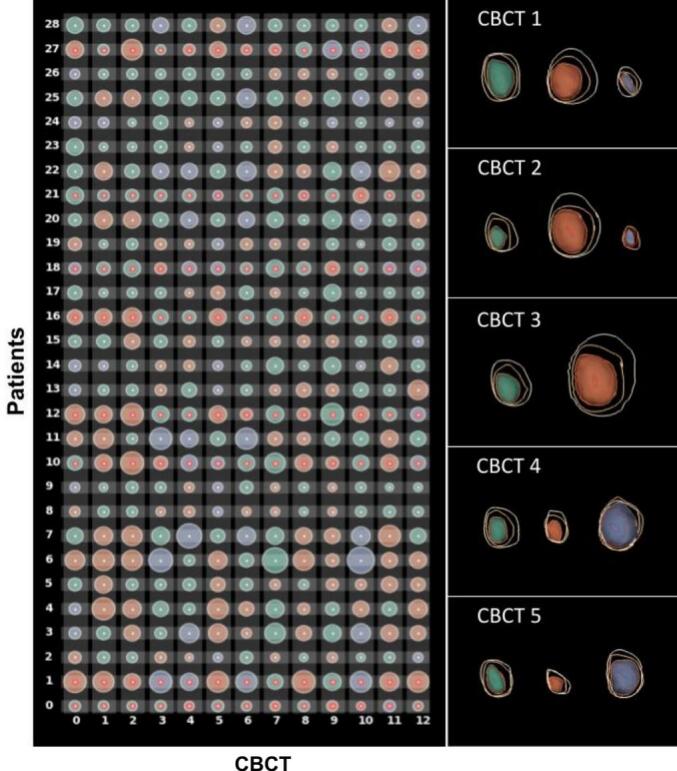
Contingency matrix (left) representing bladder volume changes and cluster assignment for all patients (rows), for all CBCTs (columns). Here, each bladder has been abstracted to a circular glyph: the color indicates the cluster assignment (Cluster 1 – orange, Cluster 2 – green, or Outliers – purple), the size indicates the volume of the bladder, and red dots indicate presence of GU ≥Grade late effects. Example of the three patient clusters (Right) computed for the first four CBCTs, where color indicates cluster assignment and the contours indicate the coverage levels (100 %, 75 %, 50 %, 25 %, 10 % going outwards from the center of the bladder). The 50 % coverage level is additionally indicated by a 3D surface rendering of the bladder (i.e., the colored green, orange, or purple surface).
